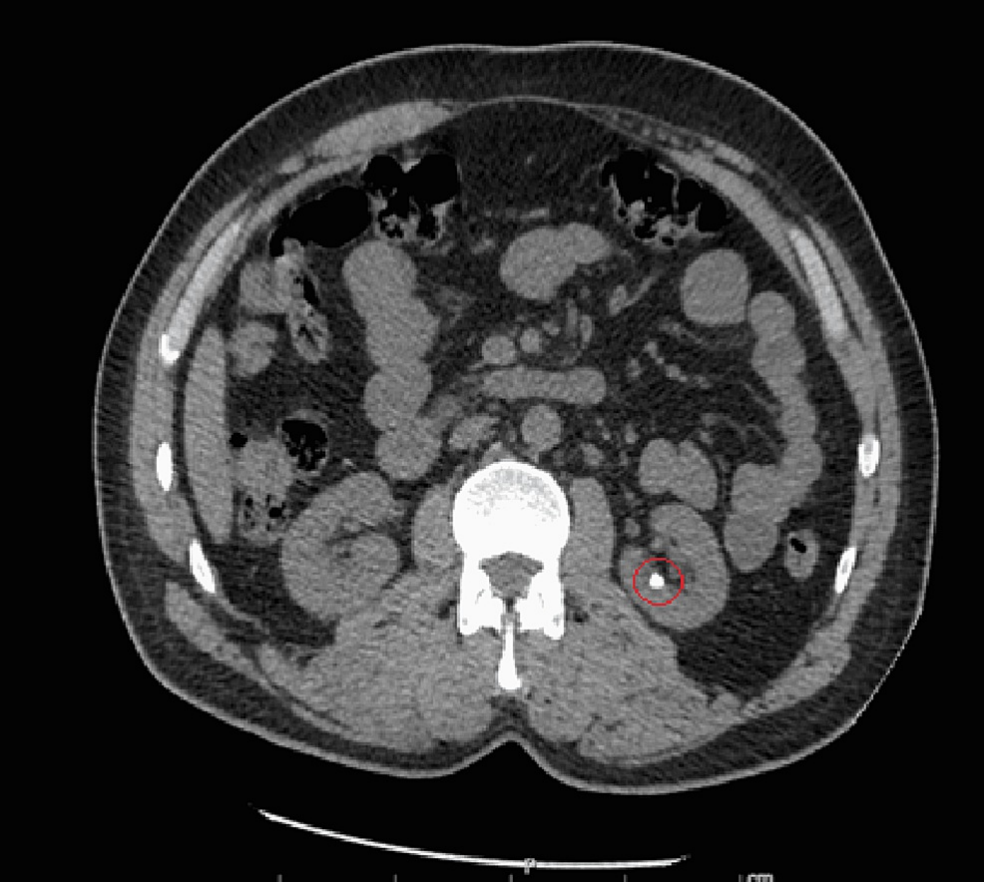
Axial view of CT abdomen pelvis without IV contrast. Red circle marking 6 mm non-obstructing left renal stone.
